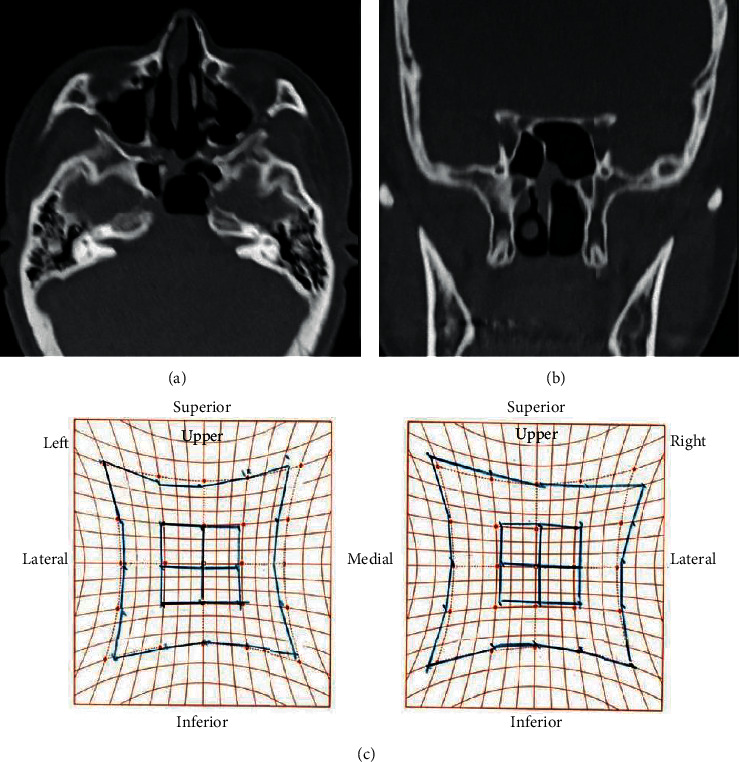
Postoperative evaluations. (a), (b) Postoperative computed tomography (plain) imaging of the paranasal cavity with a diminished sphenoid sinus mucocele, and (c) Hess screen test showing improvement in the abduction disturbance.
